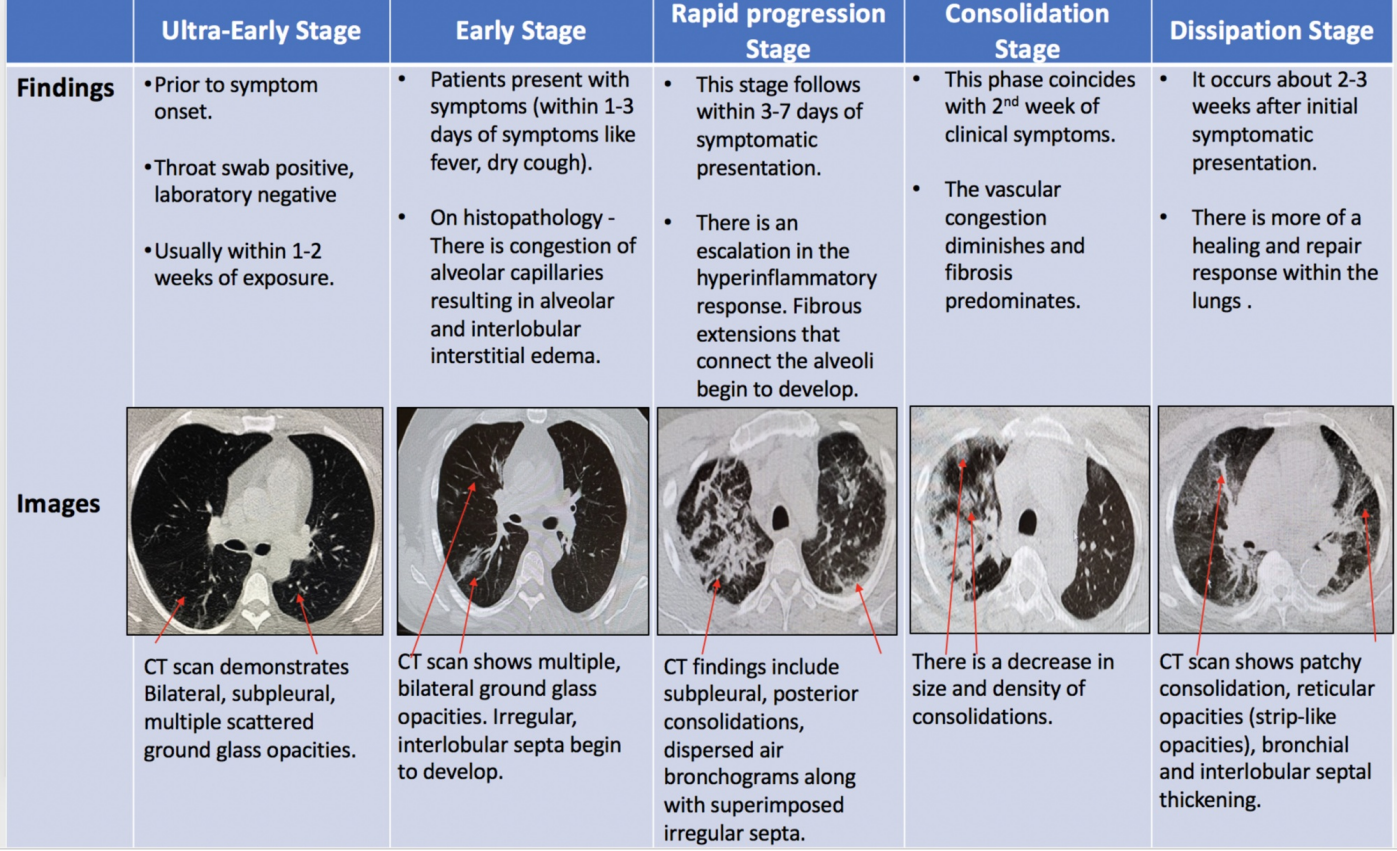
CT scan stages in patients with COVID-19
